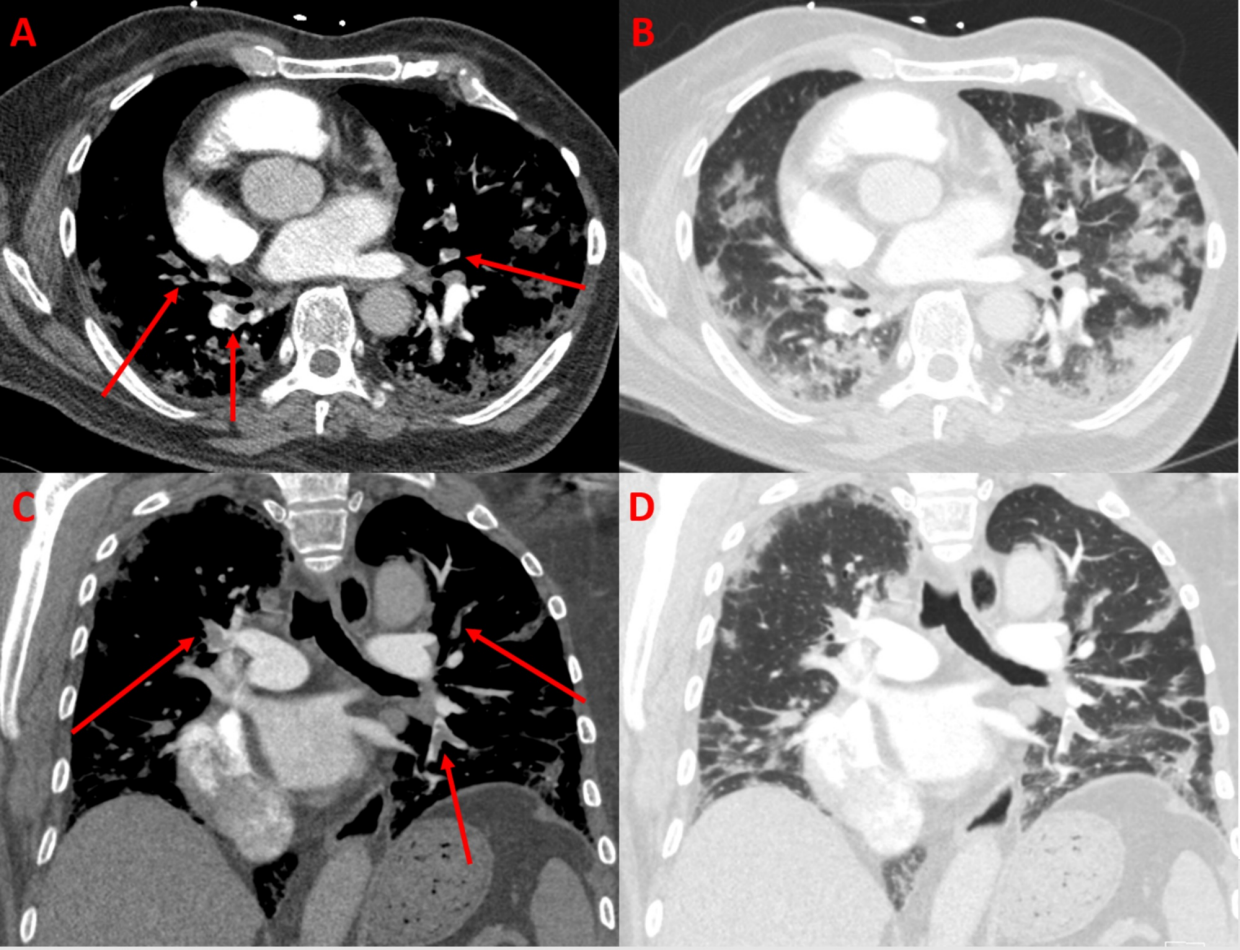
CTA-PE protocol of the chest with axial (A, B) and coronal (C, D) views show numerous filling defects in the bilateral lobar and segmental pulmonary arteries (arrows) consistent with pulmonary emboli. Lung windows show peripheral predominant ground-glass and consolidative opacities (B, D). CTA-PE, CT angiogram pulmonary embolism
